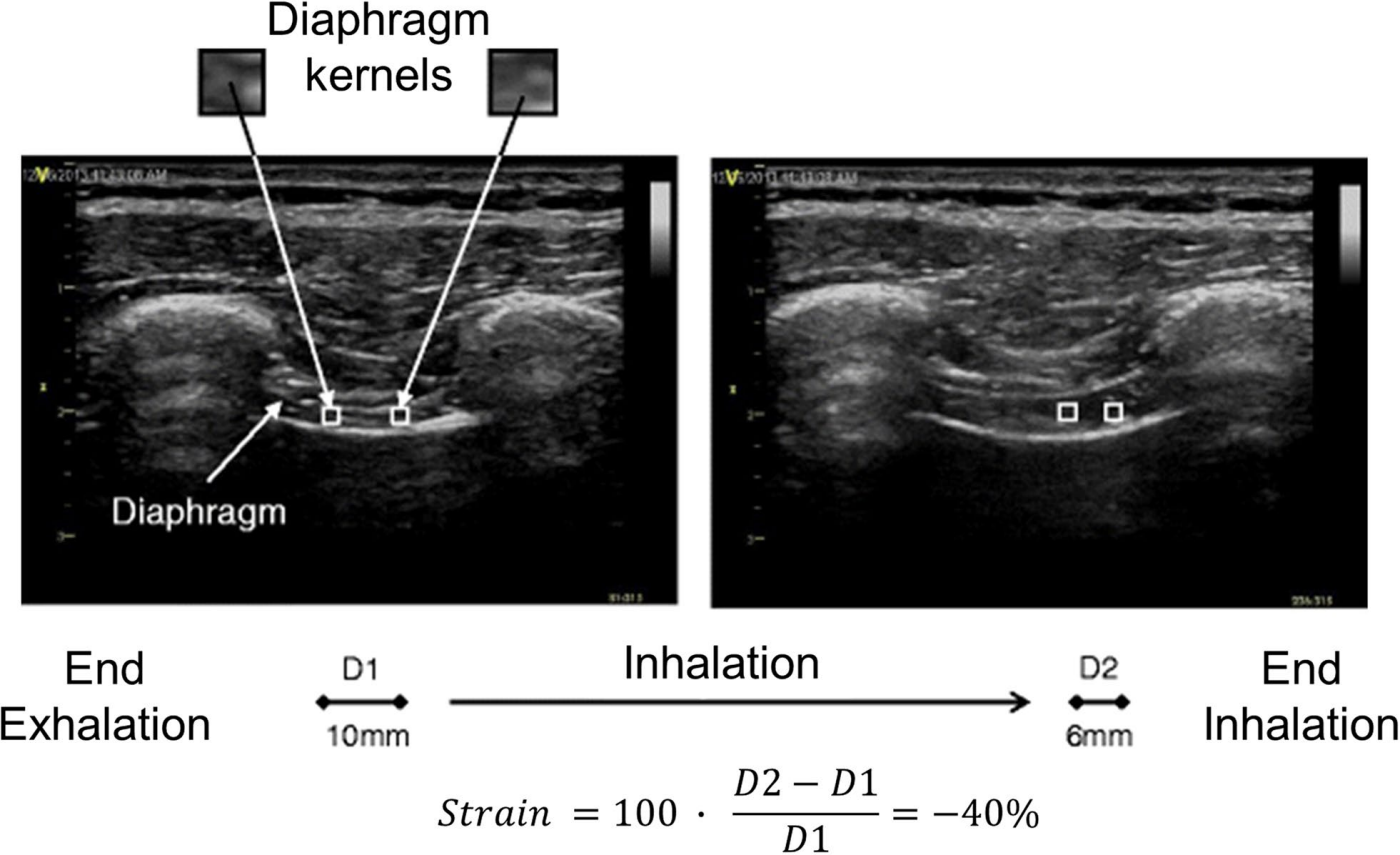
Two-dimensional speckle tracking imaging of the diaphragm’s zone of apposition at end-exhalation (*left panel*) and end-inhalation (*right panel*). The image of the diaphragm has a granularity caused by an inherent ultrasound artifact known as speckle. A cluster of speckles form a kernel. The stronger the contraction of the diaphragm, the closer kernels come together (strain). With speckle-tracking software, it is possible to quantify the strain of the diaphragm as: 100 multiplied by the difference of the distance between two representative kernels at end-inhalation (D2) minus the distance between the same kernels at end-exhalation (D1) divided by D1. In the example above, the distance between two representative kernels at end-exhalation (*left panel*) is 10 mm (D1) and at end-inhalation (*right panel*) 6 mm (D2), yielding a strain of -40%. Reproduced under Open Access Creative Commons License: Orde et al. BMC Anesthesiol 2015;16(1):43
